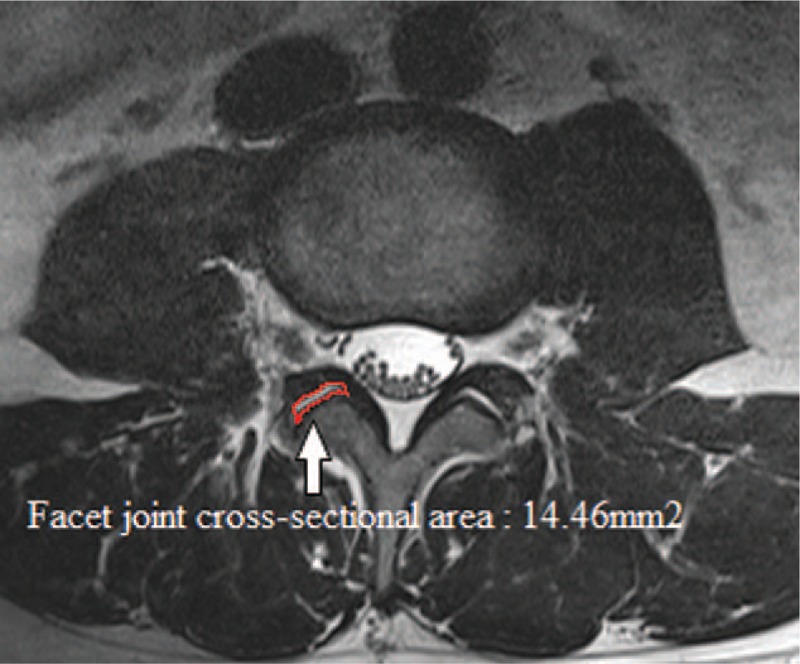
Measurement of the facet joint area on MRI at the L4-L5 level.
